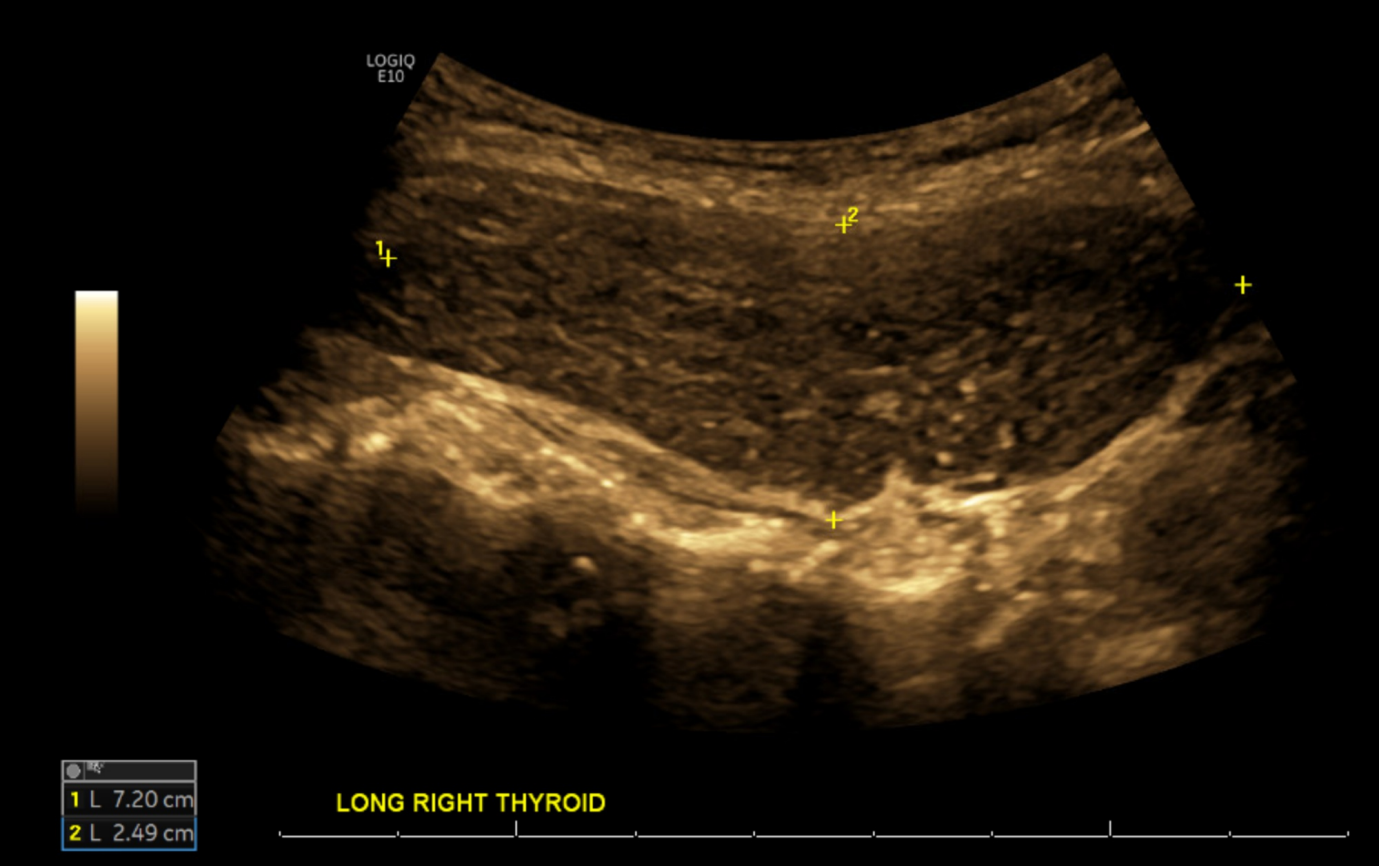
Thyroid ultrasonography, longitudinal view: enlarged, heterogeneous right thyroid lobe measuring 7.2cm x 2.49cm
